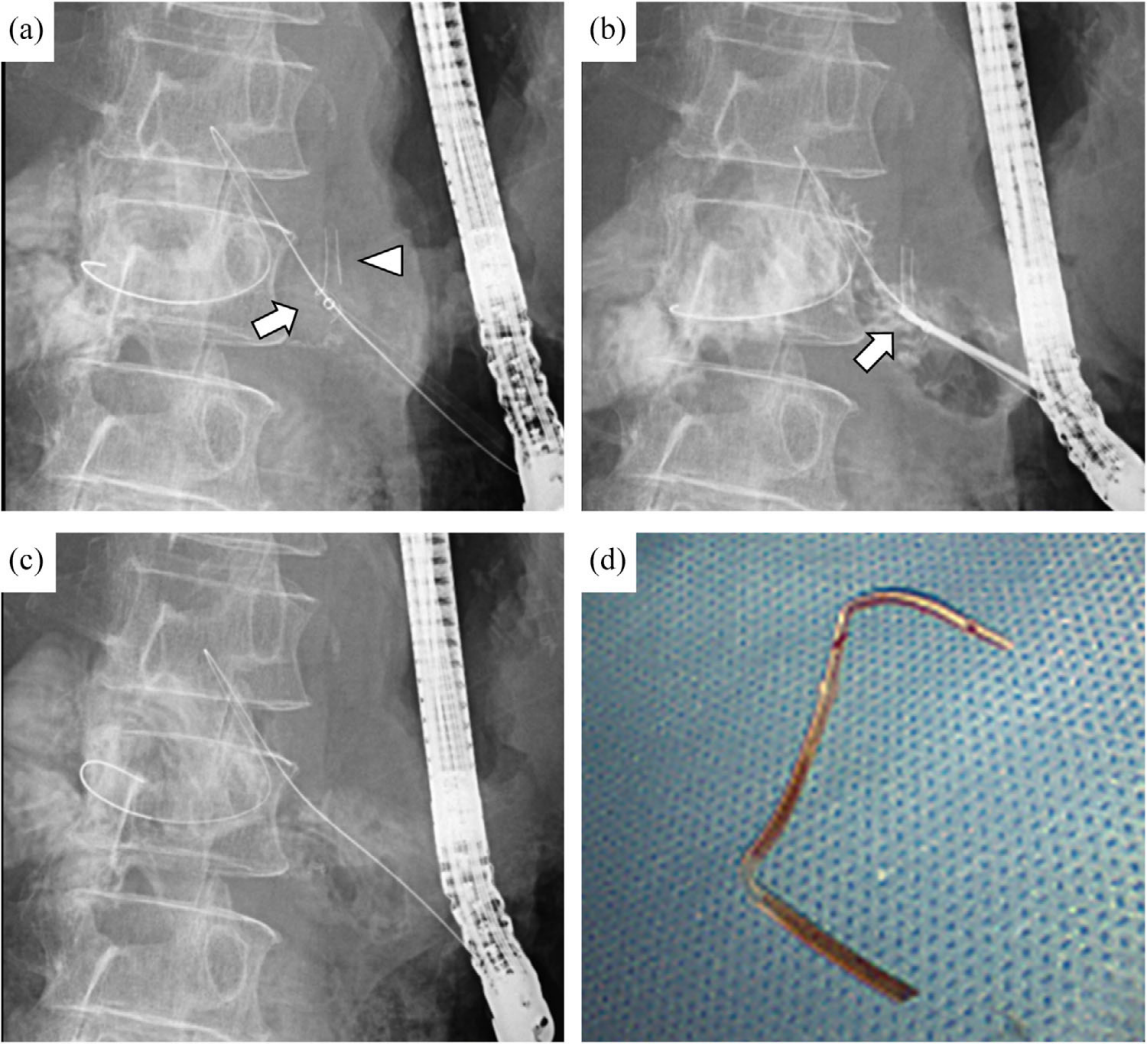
Radiographs showing the removal of a stent that had migrated into the pancreatic duct. (a) An endoscopic introducer (arrow) was placed near the internal stent (arrowhead) within the pancreatic duct. (b) Biopsy forceps (arrow) were inserted into the endoscopic introducer to directly grasp the internal stent. Both the introducer and the stent were retracted together. (c) The internal stent was successfully removed without any remnants. (d) Photograph of the removed internal stent.
